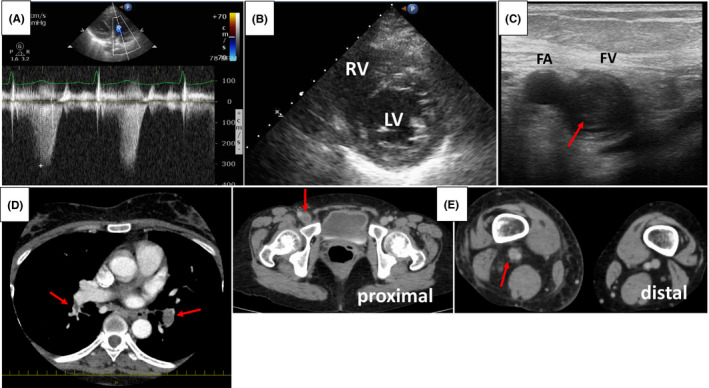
Findings from echocardiography, lower extremity ultrasonography, and computed tomography regarding venous thromboembolism. Echocardiography shows a mildly increased tricuspid regurgitation peak gradient (A) and no evidence of a D‐shaped left ventricle in the short axis view (B). RV: right ventricle. (C): Lower extremity ultrasonography reveals a partially floating thrombus (red arrow) with low‐to‐isometric echogenicity in the proximal femoral vein. FA: femoral artery, FV: femoral vein. (D, E): Contrast‐enhanced computed tomography reveals bilateral pulmonary artery embolic thrombi (red arrows, D) and deep vein thrombosis extending from proximal to distal in the right femoral vein
